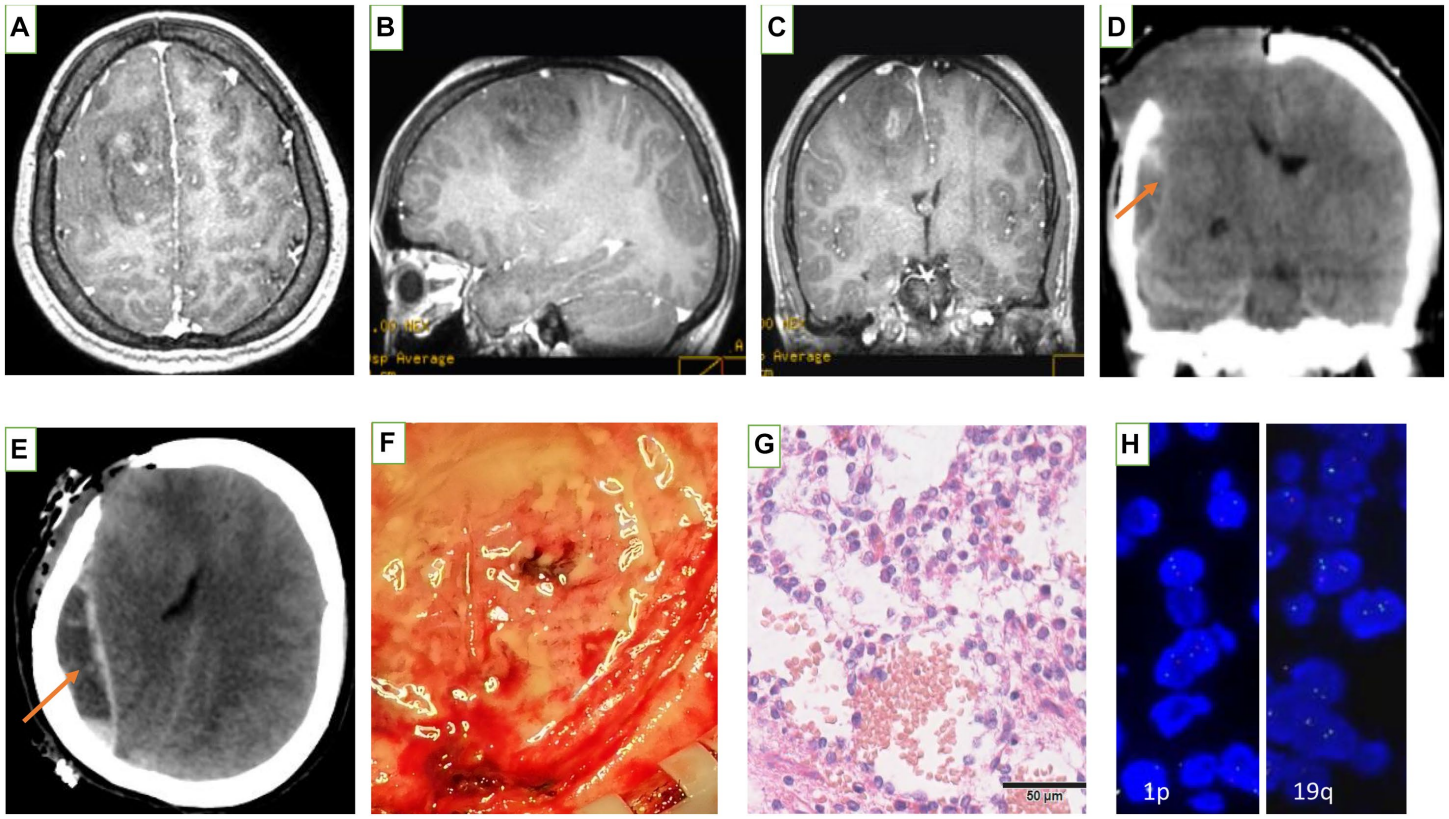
Images obtained from the preoperative cranial MRI: the right frontal lobe space-occupying lesions. (A) axial T1 enhanced, (B) Sagittal T1 enhanced, and (C) Coronal T1 enhanced. (D,E) Emergency cranial CT during the operation: found the low-density of the epidural behind the surgical area. (orange arrow). (F) Photo of the epidural during decompressive craniectomy: gelatinous substance in the epidural area. (G,H) Pathological examination confirm the right frontal lobe mass is anaplastic oligodendroglioma, IDH mutated, 1p/19q codeleted, (WHOIII): scale bar 50um.
